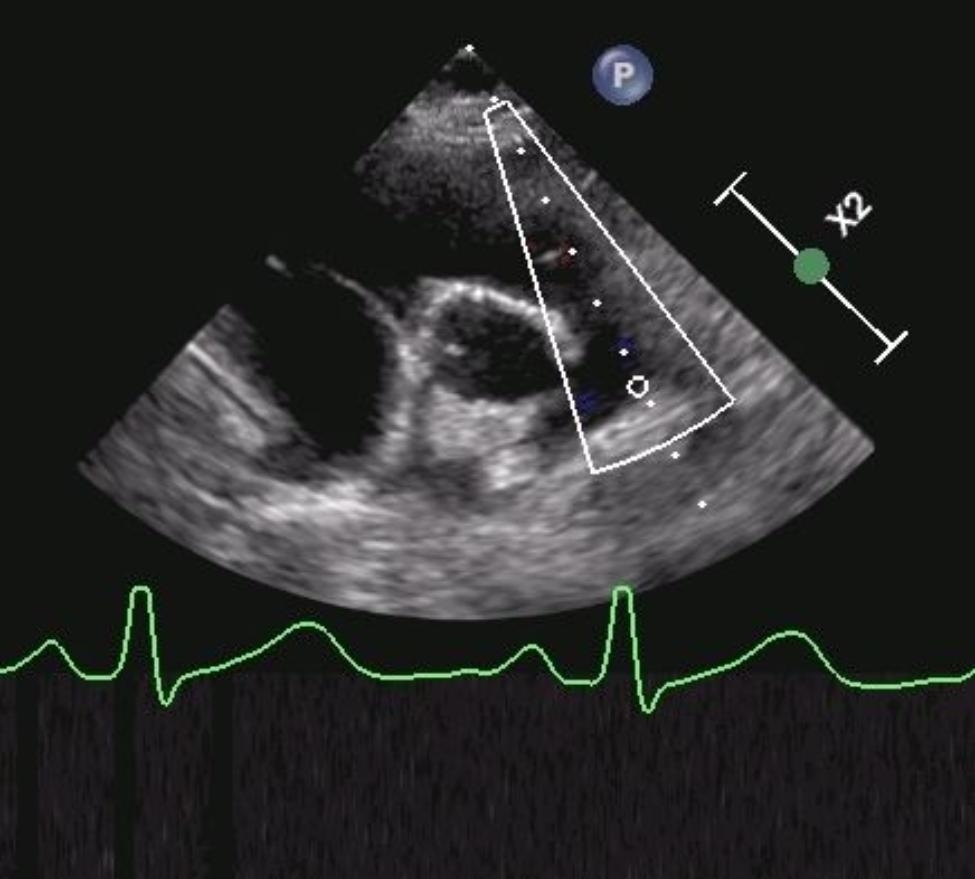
Echocardiography showing mild right sections dilatation
